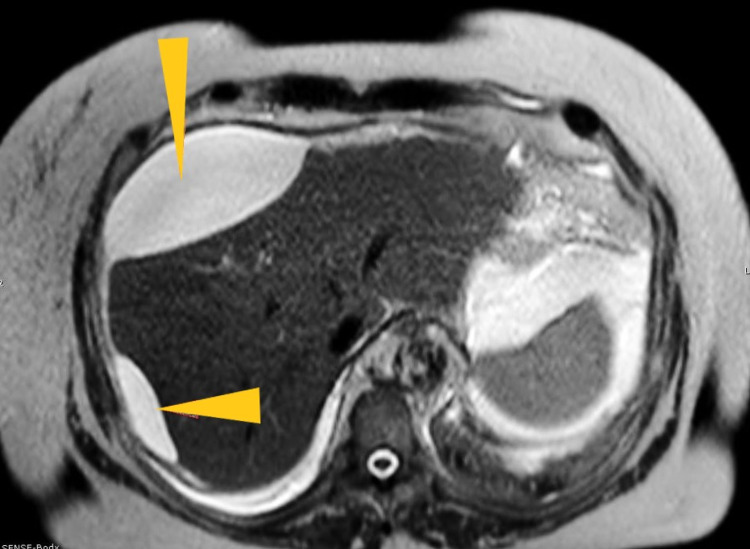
Magnetic resonance image of the biliary tree showing perihepatic bile ascites causing mass effect on the liver.
